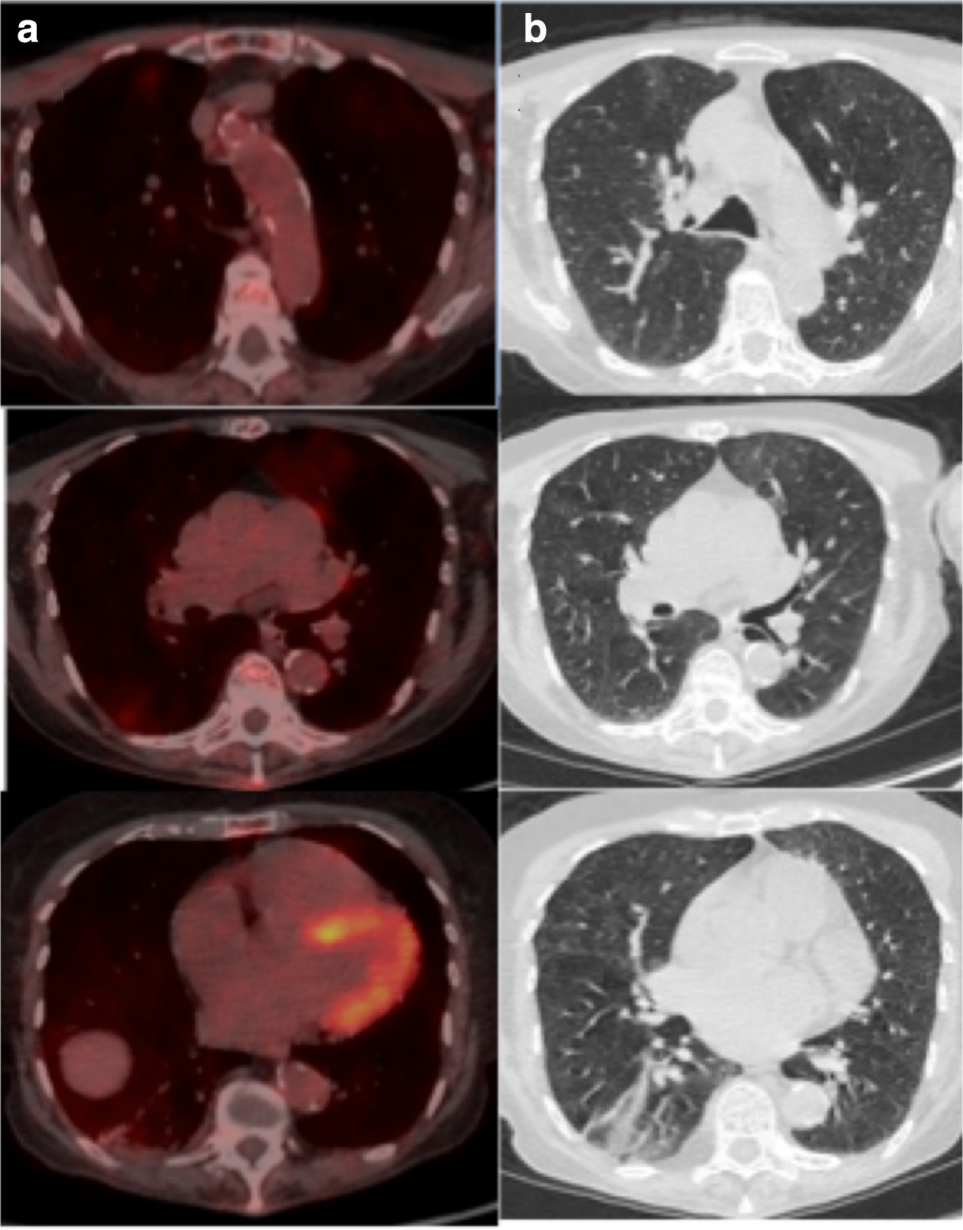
(a) Axial fused thoracic ^18^FDG-PET/CT, (b) axial low-dose CT thorax. The images demonstrate hypermetabolic GGO in three lobes with plugging and atelectasis in the lower lobes. ^18^FDG-PET, 18-fludeoxyglucose-positron emmision tomography; GGO, ground glass opacity.
